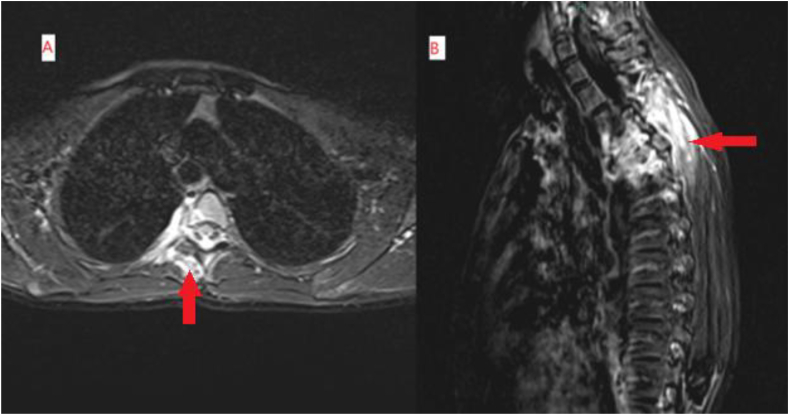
Magnetic resonance imaging (MRI) of the thoracic spine demonstrating a focal lesion centered at the right T4-5 facet joint, with surrounding paravertebral muscle edema (red arrows), without epidural extension or spinal cord compression. (A: axial cut; B: sagittal cut).
